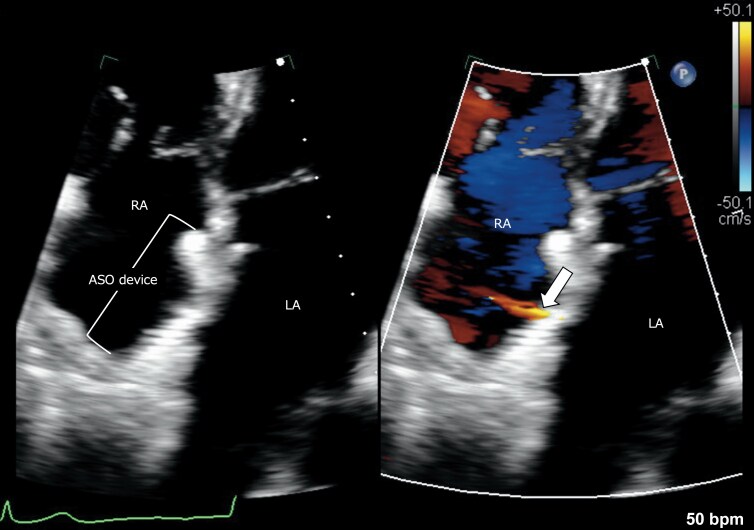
Residual shunt on transthoracic echocardiography. A transthoracic echocardiographic image delineating a residual interatrial shunt within 2 months after ASO device puncture. The residual shunt flow (arrow) was minimal in size and haemodynamically insignificant. ASO, atrial septal occluder; LA, left atrium; RA, right atrium.
